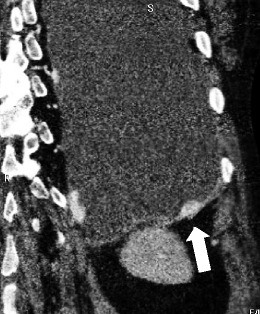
Stage II of MM; contrast-enhanced CT scan: coronal view. Focal pleural enhancing thickening that infiltrates the left hemidiaphragm (*thick arrow*)
